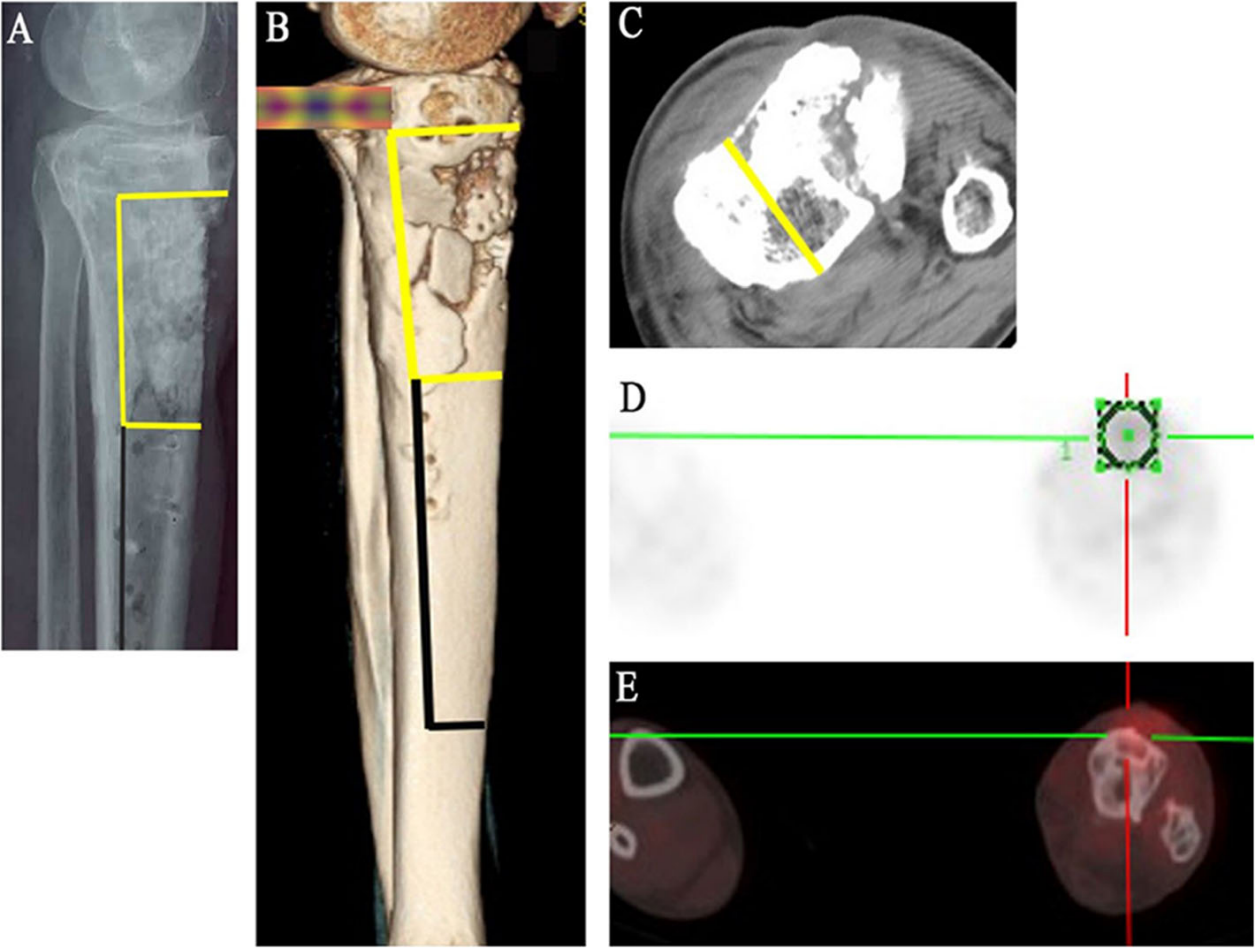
a–g A 60-year-old man with chronic osteomyelitis of the left tibia. Imaging examinations are taken to assist in determining the extension of infection and resection. a A preoperative lateral radiograph shows the extension of the infection that compromises the proximal diaphysis and margins of resection (yellow line) and corticotomy (black line) is determined. b, c The CT scans show the extension of bone destruction and margins of resection (yellow line) and corticotomy (black line) is determined on lateral (3D) and coronal images. d, e The PET-CT scan shows increased uptake in the area of infection
